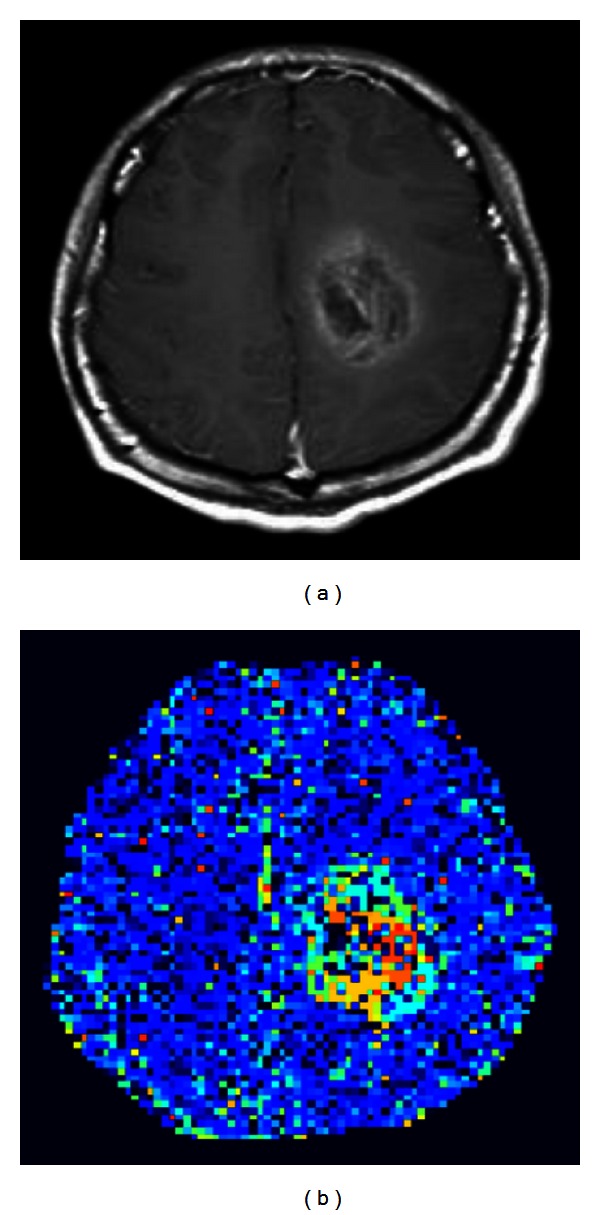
An anaplastic oligodendroglioma, WHO grade III, in the left frontal lobe shows contrast enhancement and leakage on T1-weighted image (a) and *K*
